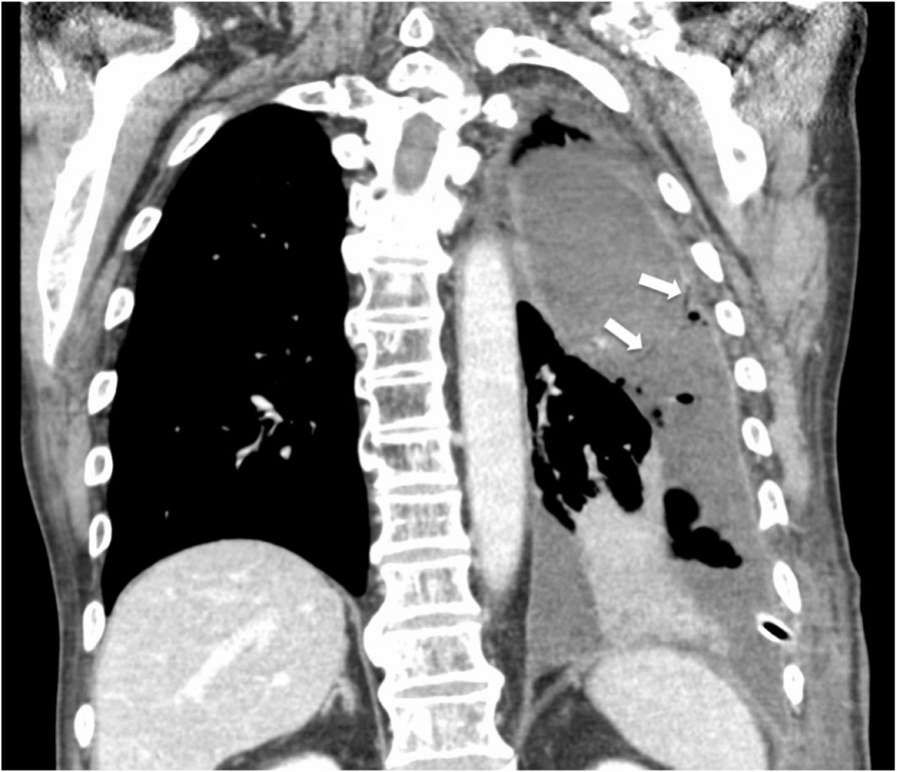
Preoperative chest computed tomography showing poor lung re-expansion, loculated pleural effusions, and a residual extrapleural hematoma with extrapleural fat signal (white arrow) after placement of the second chest tube
